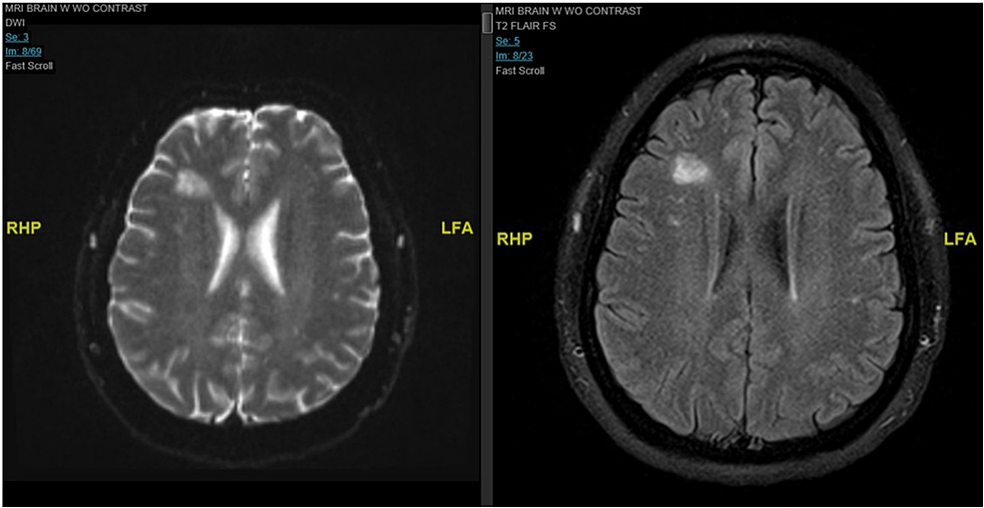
T2 DWI (left) and FLAIR MRI (right) MRI demonstrating a globular focus of increased signal intensity within the right frontal lobe juxtacortical white matter measuring 14 x 17 mm transaxially. DWI: diffusion-weighted imaging; FLAIR: fluid-attenuated inversion recovery
